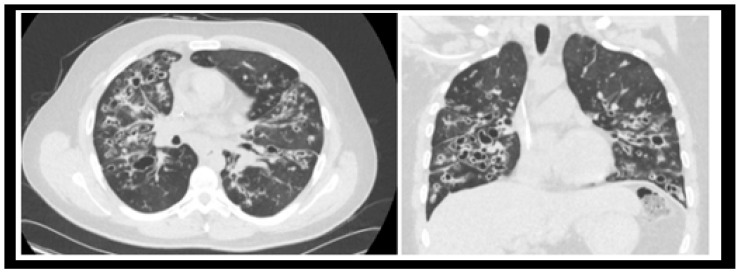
CT radiograph of a patient with advanced CF lung disease demonstrating extensive bronchiectasis and mucoid impaction. Patient was heterozygous for F508del with a forced expiratory volume in 1 s (FEV1) of 0.74 L/22% predicted, history of several pulmonary exacerbations per year, chronic hypoxemia and hypercapnia, and severe pulmonary hypertension. Highly effective modulator therapy, elexacaftor/tezacaftor/ivacaftor, had not yet been approved at the time when the patient required a bilateral lung transplantation.
